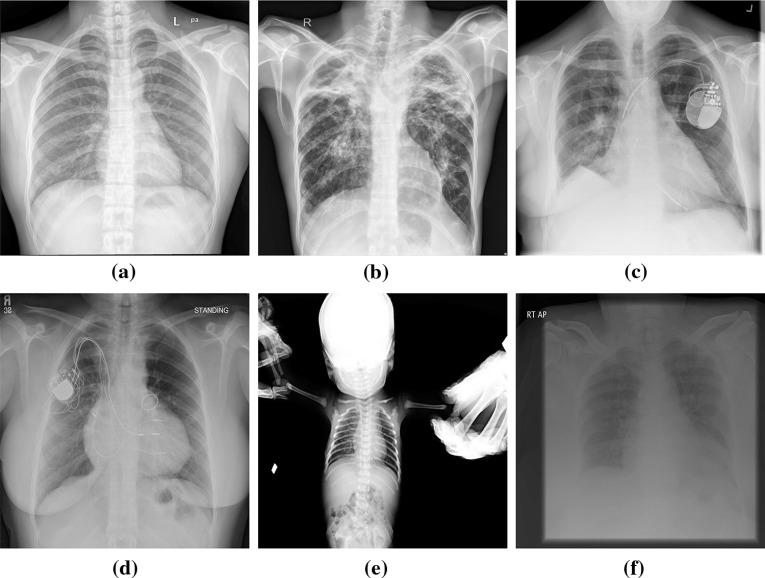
a A healthy lung. b–f Challenges for segmenting lung regions: b large variance of pixel values at apex due to pathology (bilateral tuberculosis with multiple cavitations), c a cardiac pacemaker, right pleural thickening, and strong breast tissue on the clavicle region of left lung, d variation of the lung appearance due to varying heart dimension, cardiac pacemaker on the left, and strong breast tissue on the clavicle regions, e image noise in pediatric CXR such as hands and patient’s head; small lung area, f an under-penetrated radiograph
